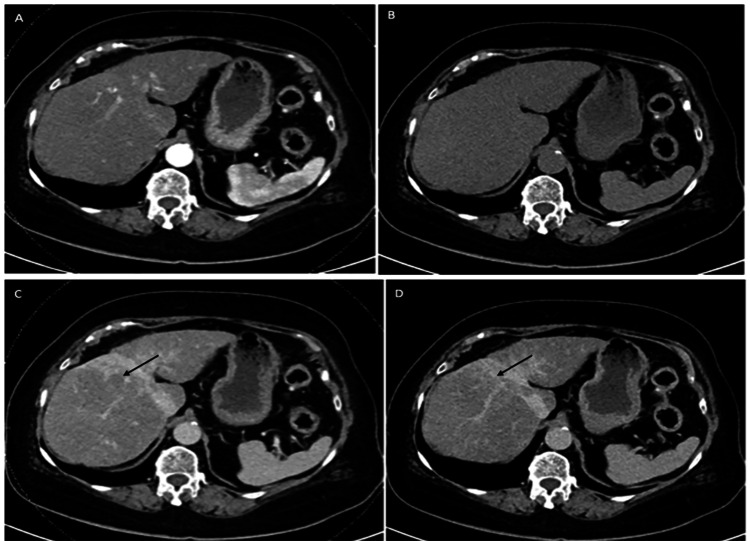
Contrast-enhanced CT liver (November 2022) The mass appears isodense to the liver on the non-contrast image (A). No significant enhancement is appreciated on the arterial phase image (B), nor does it demonstrate appreciable washout on the PV (C) or delayed images (D) CT: computed tomography; PV: portal venous
